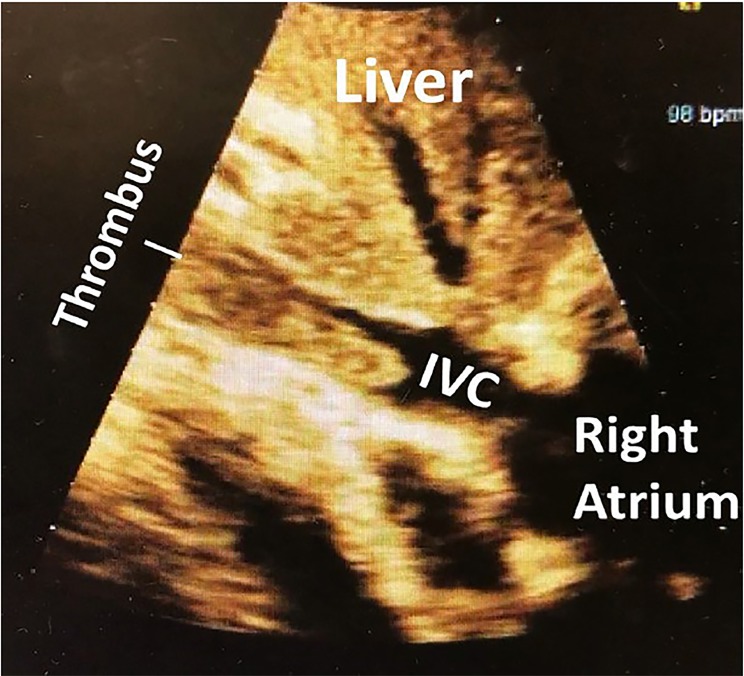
Point-of-care ultrasound phased array probe in the subxiphoid orientation revealed a thrombus situated in the inferior vena cava (IVC).
